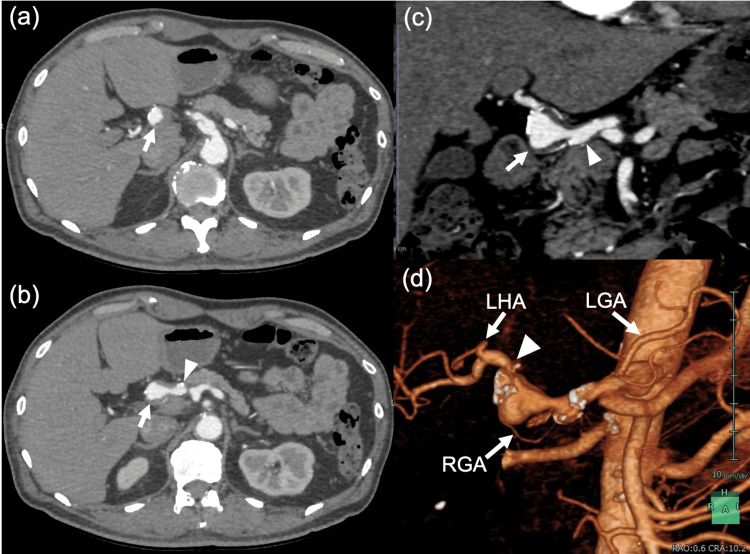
Initial contrast-enhanced CT and volume-rendered images at diagnosis (a, b) Axial arterial-phase contrast-enhanced CT images (b is 5 mm caudal to a). (c) Coronal arterial-phase contrast-enhanced CT image. An irregular long-segment aneurysmal ectasia is seen from the common hepatic artery to the proper hepatic artery. A focal saccular component measuring up to 17 mm in maximum diameter is seen (arrow). A dissection-related change is suspected immediately proximal to the saccular component (arrowhead), and mural thrombus is visible within the saccular component. Dissection-related change is also suspected in the celiac trunk. (d) Volume-rendered three-dimensional reconstruction from the same CT dataset. The image demonstrates the long-segment aneurysmal morphology and its anatomic extent from the common hepatic artery to the proper hepatic artery. The left hepatic artery (LHA) branches immediately distal to the long-segment aneurysmal segment. A collateral pathway from the left gastric artery (LGA) to the right gastric artery (RGA) is suggested, and the RGA appears to join the distal proper hepatic artery near the margin of the saccular component (arrowheads).
